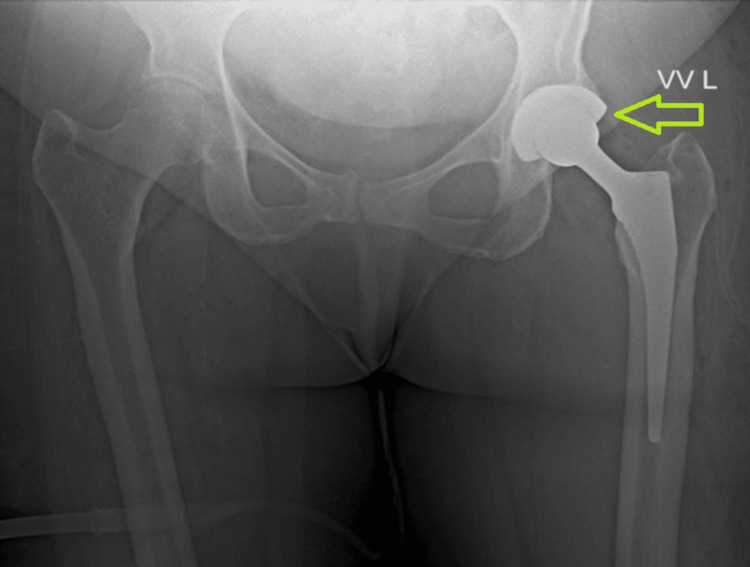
Postoperative radiograph after component revision. Anteroposterior plain radiograph of pelvis with both hip joints showing the newly implanted components, including the modular femoral head and polyethylene liner. The components are properly aligned, with no signs of dislocation. Green arrow showing well reduced left hip joint.
